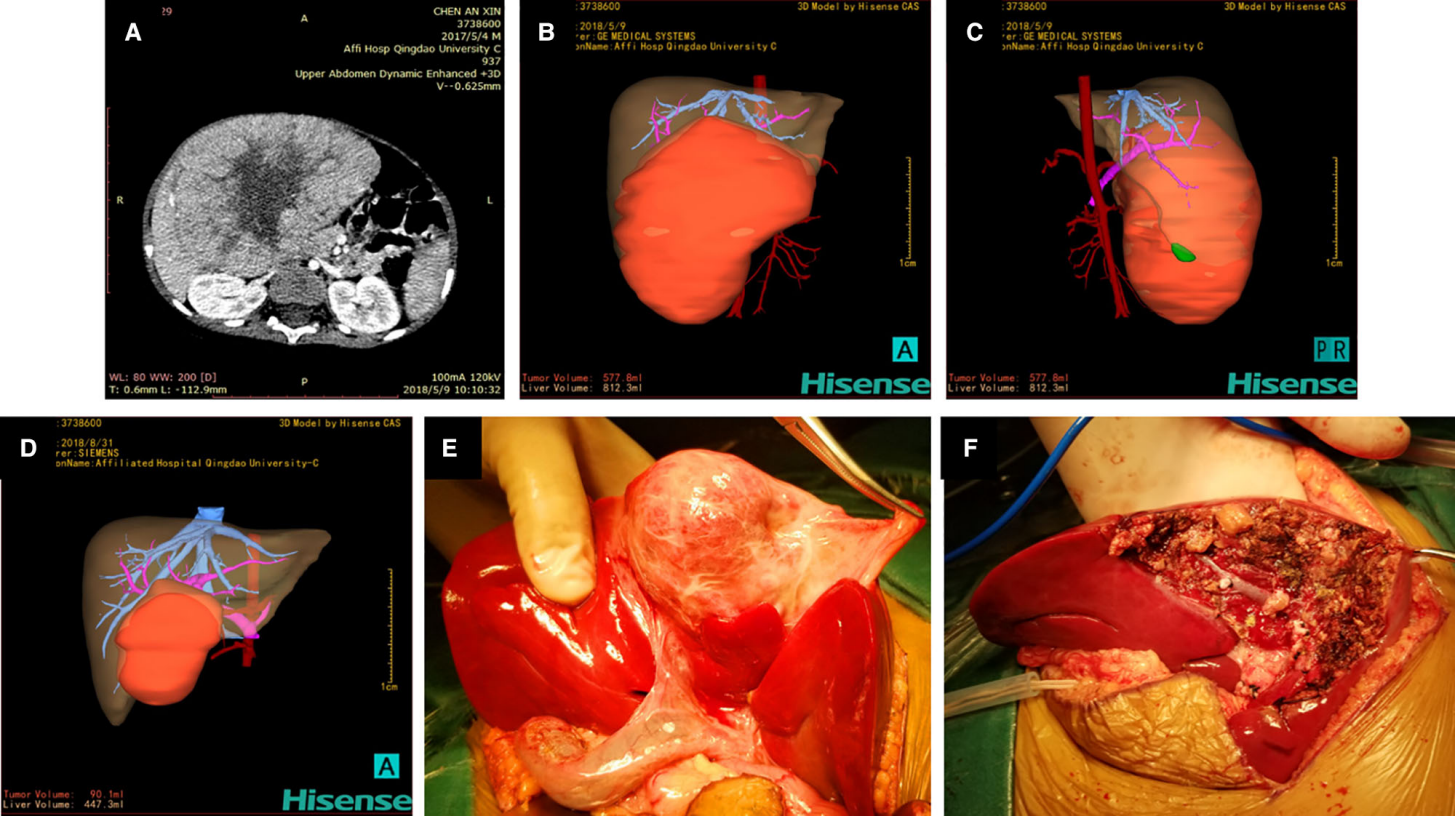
Hisense computer-assisted surgery system was used for three-dimensional reconstruction of hepatoblastoma (Case 9) at each stage. (A) Two-dimensional CT images of hepatoblastoma before chemotherapy. (B, C) Three-dimensional reconstruction image of hepatoblastoma before chemotherapy showing that the tumor volume and liver volume evaluated by the software are 577.8 mL and 812.3 mL respectively. (D) Three-dimensional reconstruction image of hepatoblastoma after three cycles of chemotherapy showing that the tumor volume is 90.1 mL and the liver volume is 447.3 mL. (E) Location and size of the tumor during the operation are completely consistent with the three-dimensional reconstruction image. (F) Intraoperative images after complete resection of the tumor.
